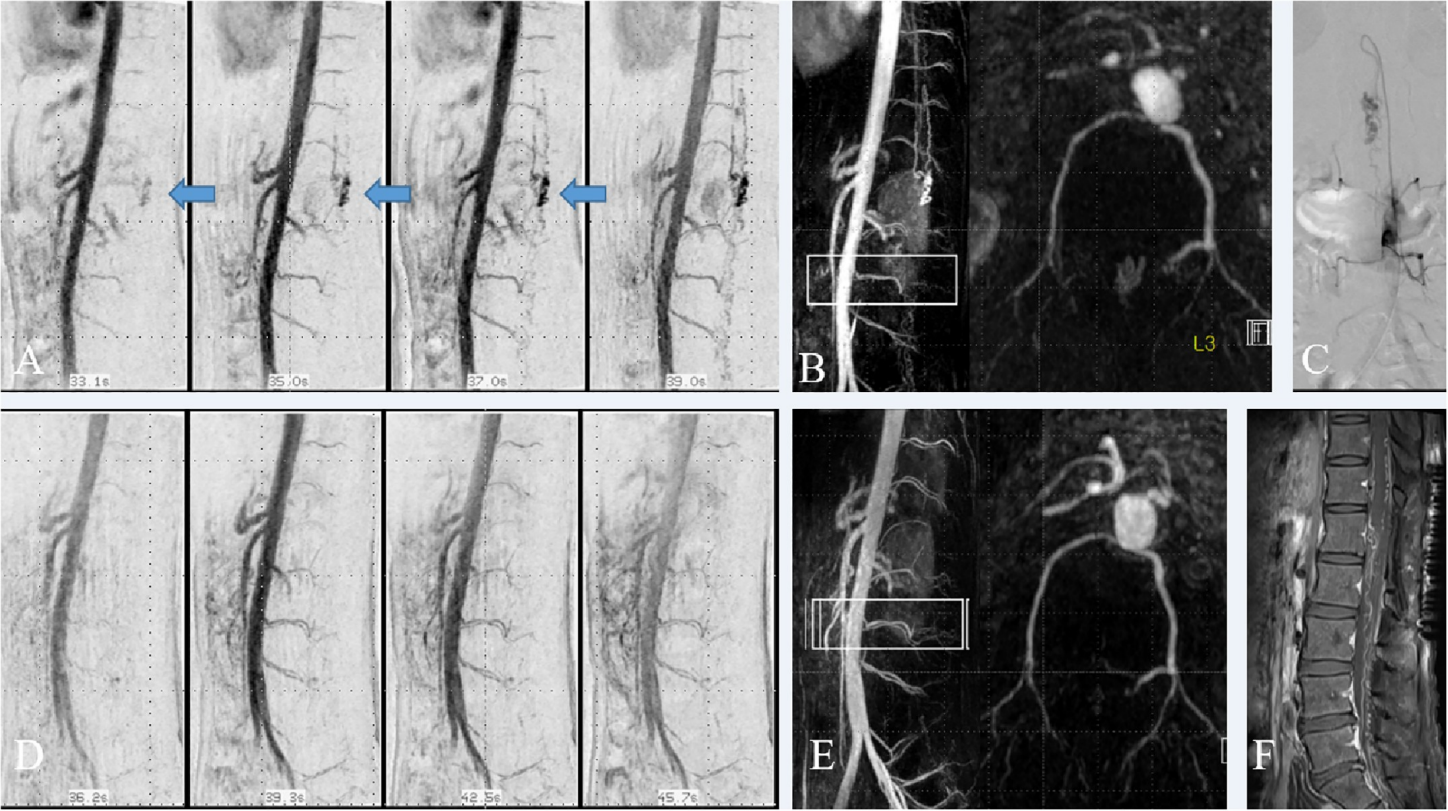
A 33-year-old man with perimedullary arteriovenous fistula (pmAVF) (patient 6). Sequential MIP images of low-dose TR CE-MRA showed early visualization of dilated venous sac (arrow) and perimedullary venous drainage (A). In addition, there were hypoplastic both 1st lumbar arteries, which were confirmed on subsequent DSA (not shown). MIP and segmented axial MIP images of single-phase HR CE-MRA (B) showed spinal AVM with feeding artery of anterior spinal artery from prominent radicular artery at left L3 level. Spinal DSA (C) confirmed spinal AVM with anterior spinal artery arising at the matched level. Follow up low-dose TR CE-MRA (D) obtained 7 days after surgery revealed obliteration of previously noted dilated venous sac. MIP and segmented axial MIP images of follow up single-phase HR CE-MRA (E) also showed obliteration of dilated venous sac, yet with still prominent radicular artery at left L3 level and several perimedullary vessels. Delayed contrast enhanced T1 weighted MR image (F) also showed contrast enhancement at the perimedullary vessels.
